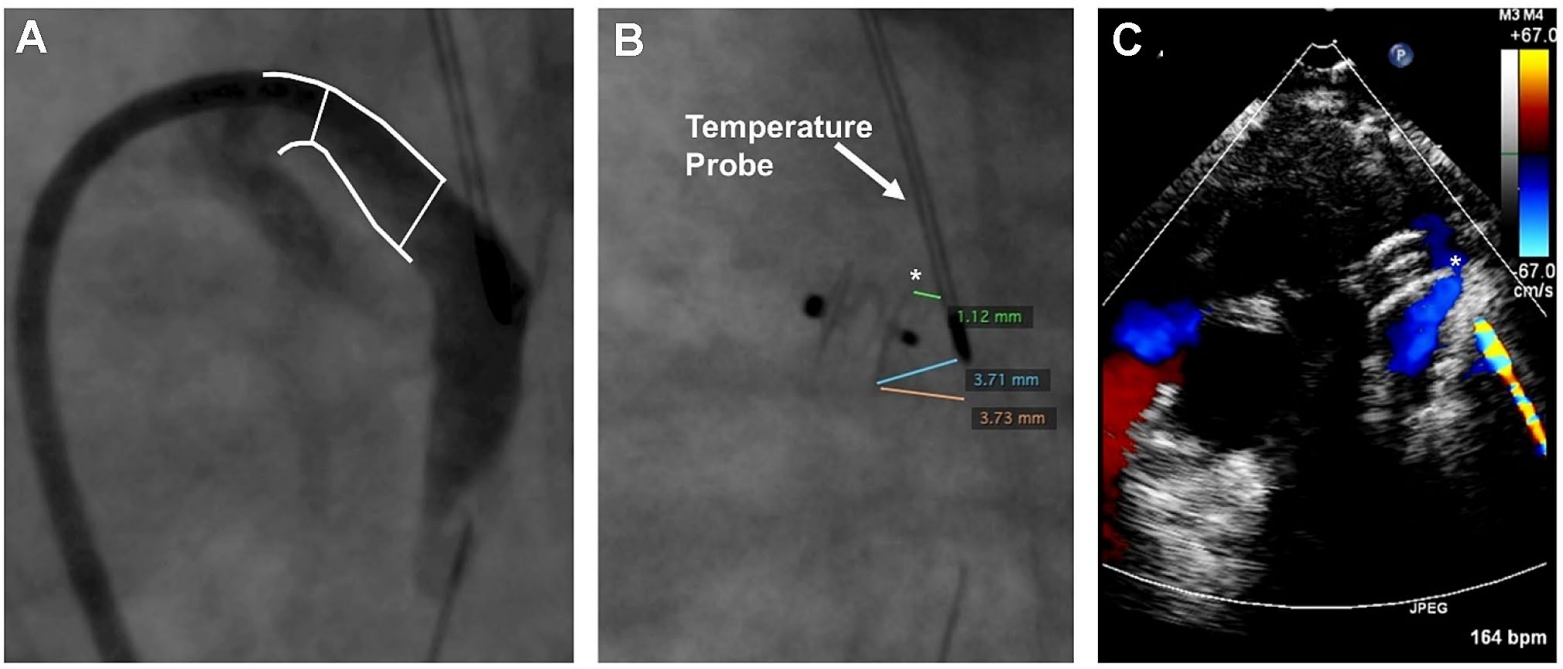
F-type PDA angiogram in 720-g infant (A) followed by closure using the Amplatzer Piccolo occluder device position relative to temperature probe (B) followed by aortic coarctation seen six hours post implant on echocardiogram (C). Asterisk (*) marks superior edge of aortic disc protruding into aorta
